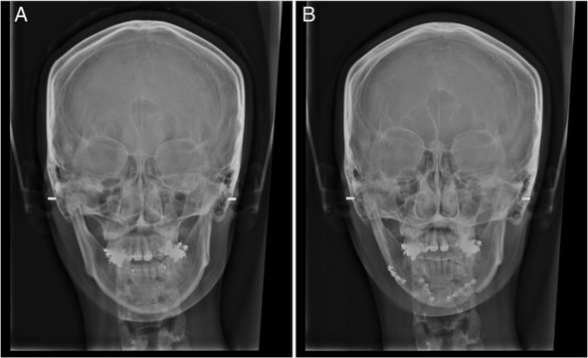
Radiographic findings of case 2: a pre-operation and b post-operation
